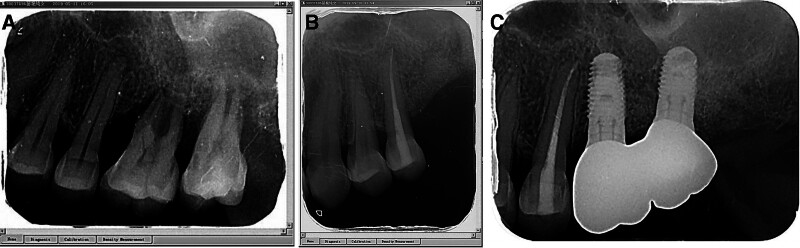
Dental radiograph of the left upper molar during root canal treatment and implant surgery. (A) Pretreatment. (B) Posttreatment of root canal treatment. (C) Five years after root canal treatment and implant surgery.
